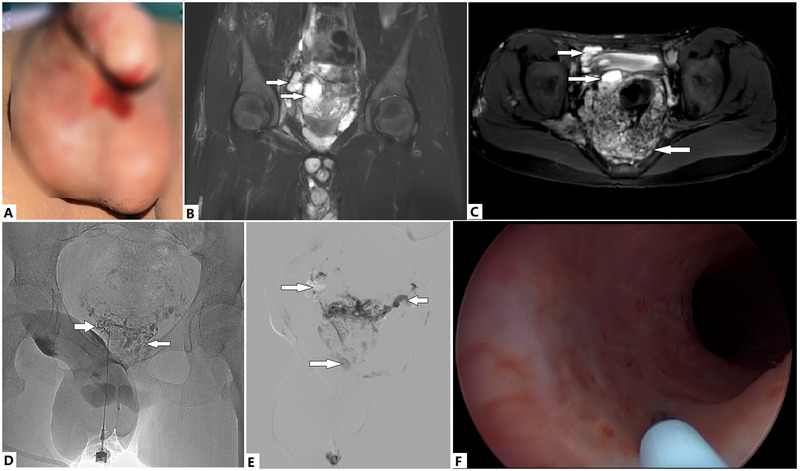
Evaluation and treatment of a 13-year-old boy with Klippel–Trénaunay syndrome (KTS) with genitourinary (GU) bleeding. At birth, the boy was diagnosed with KTS of the right lower extremity. (A) Recurrent hematuria and urethral bleeding have occurred in recent months, which have led to severe anemia and required repeated blood transfusions, despite his having undergone multiple medical treatments. (B) Venous malformations (VMs) of the prostatic and vesical venous plexuses, as revealed by T2 magnetic resonance imaging (T2 MRI) sequences (arrows). An intrascrotal VM is also visible. (C) A circumferential VM is encroaching on the vesical wall (upper rightward arrow). A cystic VM of the prostatic venous plexus is visible (lower rightward arrow). The rectal VM is also notable (left arrow). (D) VMs are directly punctured at the perineum, and vesical VM (right arrow) and prostatic VM are visible (left arrow). (E) Cystic VM of the prostatic venous plexus on MRI, which was confirmed with venography (lower right arrow). Ethanol and foam polidocanol were sequentially injected via puncture. These VMs (rightward arrows) drain into the contralateral inferior vesical vein (leftward arrow) through the malformed prostatic venous plexus. (F) Urethral bleeding is successfully managed with cystoscopic injection of ethanol at the bleeding sites. Hematuria and urethral bleeding completely resolved during the follow-up period of >1 year.
